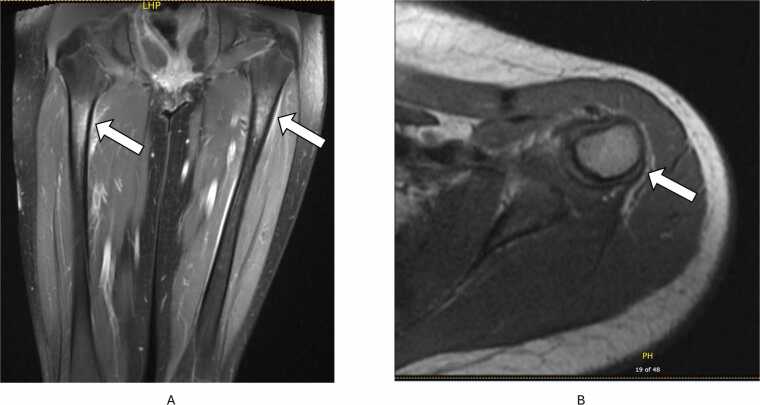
MRI demonstrating areas of marrow edema and periostitis (arrows) correlating with sites of clinical symptoms of pain. After expert review, deemed to be voriconazole-induced periostitis. (A) MRI sagittal view of bilateral femurs with marrow edema in the proximal femoral shafts with associated periostitis. (B) MRI axial view of right humerus with marrow edema and postcontrast enhancement along the medial aspect of the right proximal humeral metadiaphysis.
